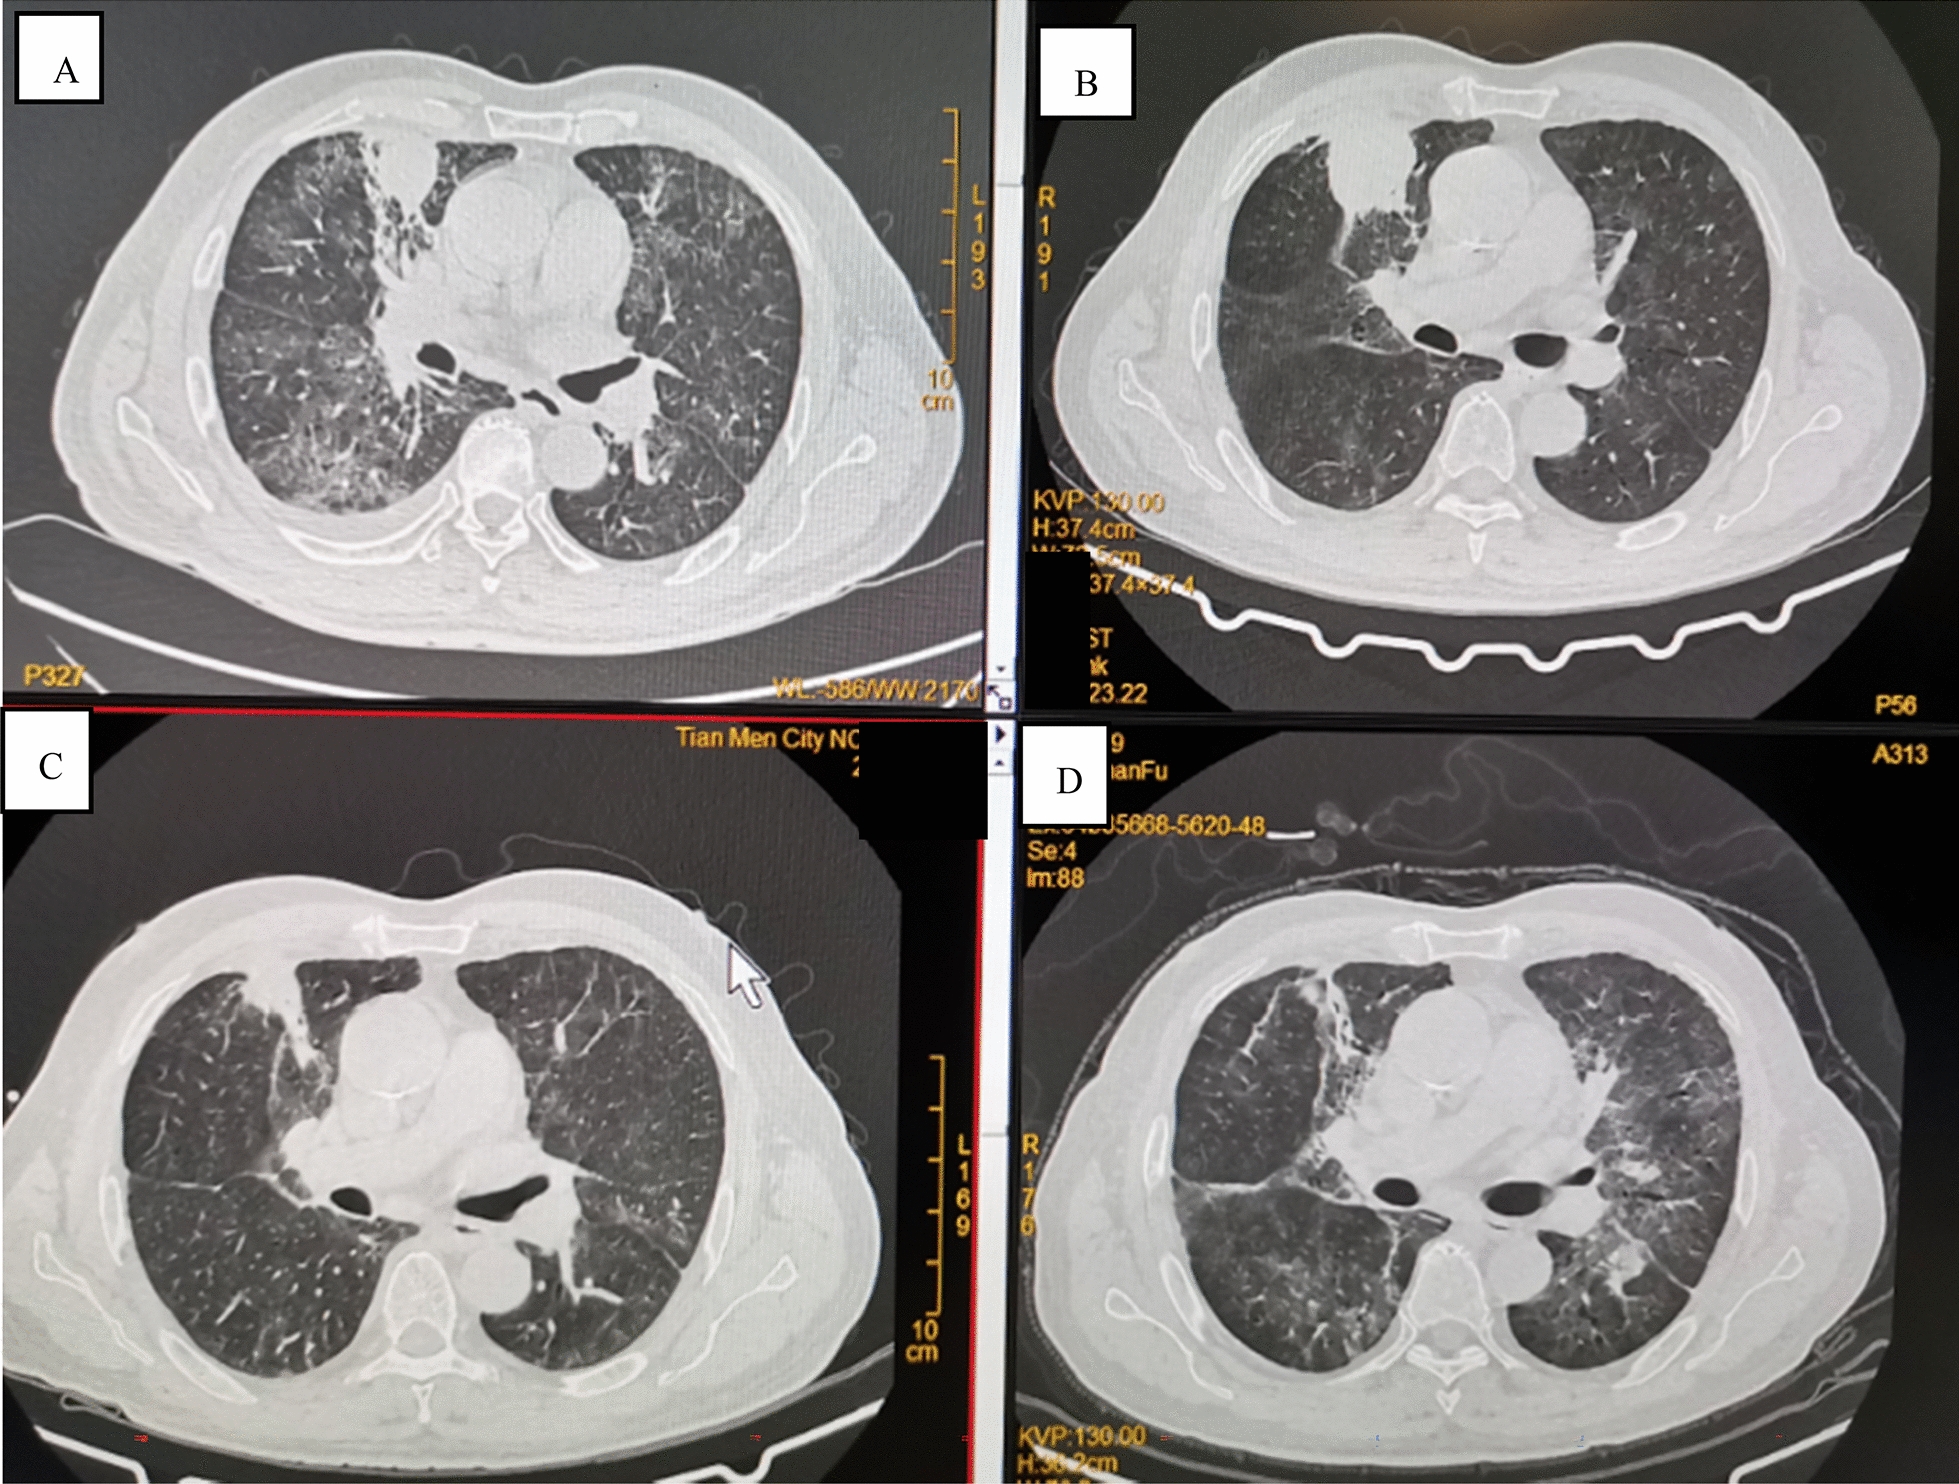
Chest CT images of the patient. A Chest CT on June 19. B Chest CT August 16. C Chest CT on September 14. D Chest CT on December 9
